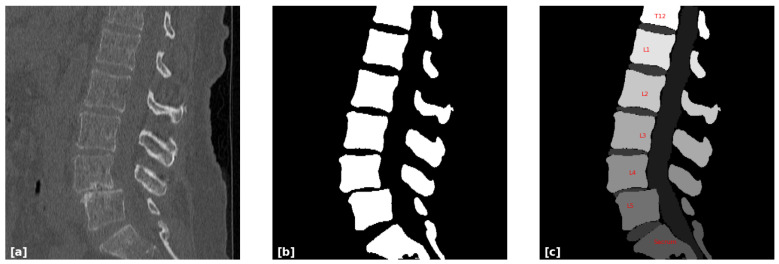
Segmentation and labeling process of a CT scan. (a) A sagittal slice from the original CT scan. (b) The 3D spine segmentation of the original CT scan on the same slice, in which all bone structures are white. (c) Labeling the different vertebrae, starting with the sacrum.
